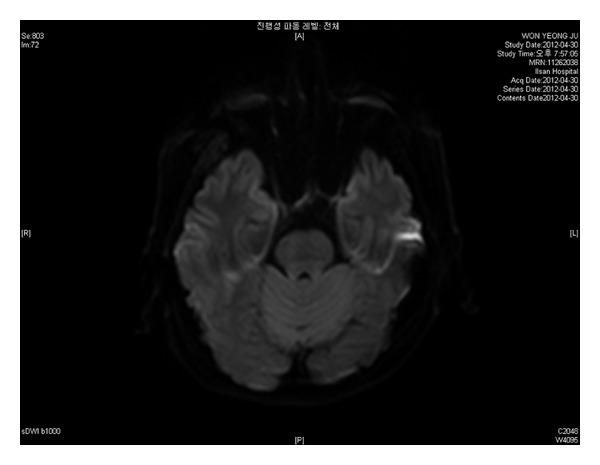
Diffusion-weighted MR image of the patient after neurological symptoms improved. Lack of diffusion restriction around the right cavernous sinus indicates that there is no residual infectious lesion.
